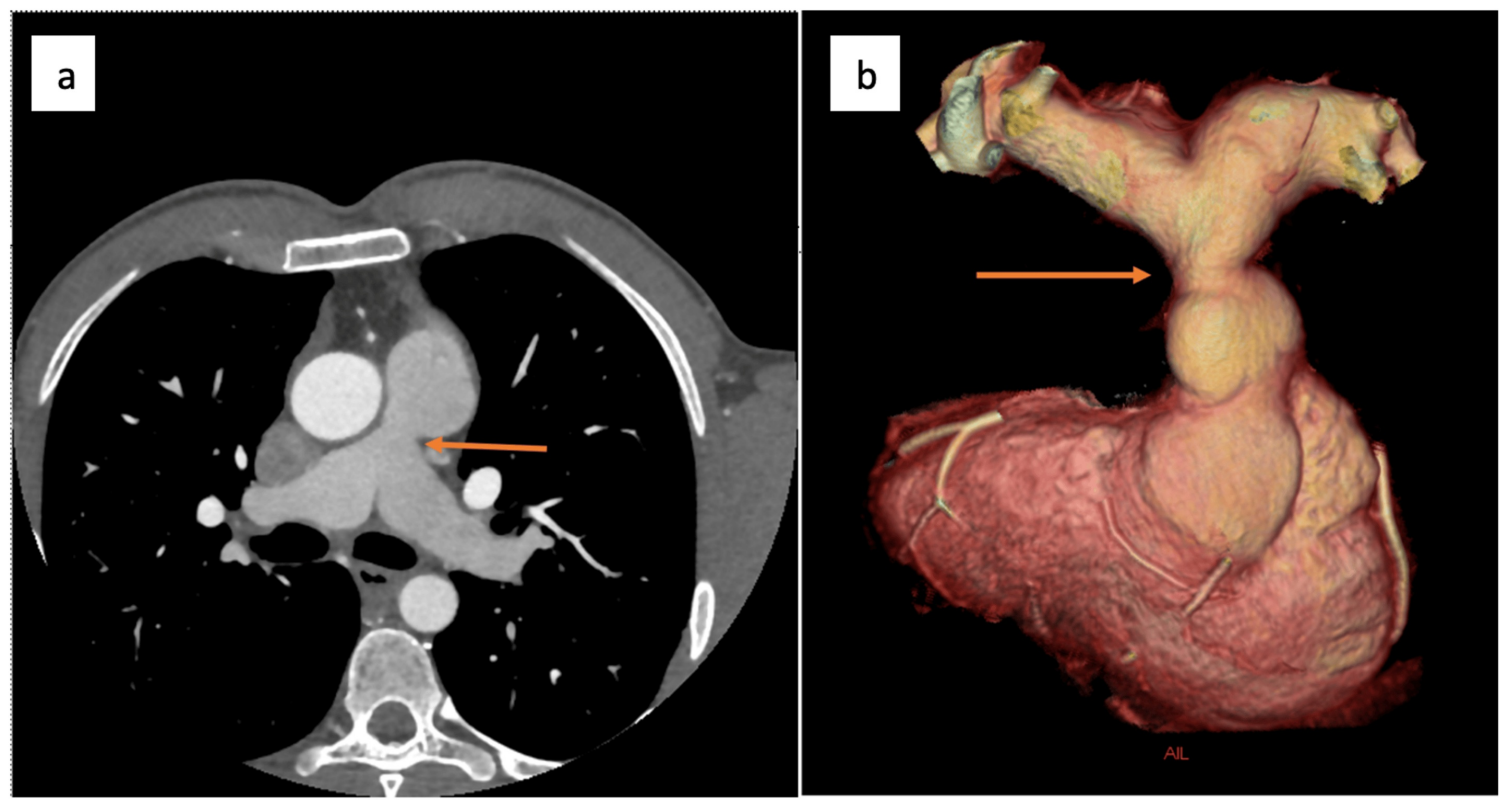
(a) Axial cardiac CT and (b) 3D volume rendering images of main pulmonary artery and side branch pulmonary arteries reveal focal supravalvular pulmonary artery stenosis (arrows) 3D: three-dimensional
